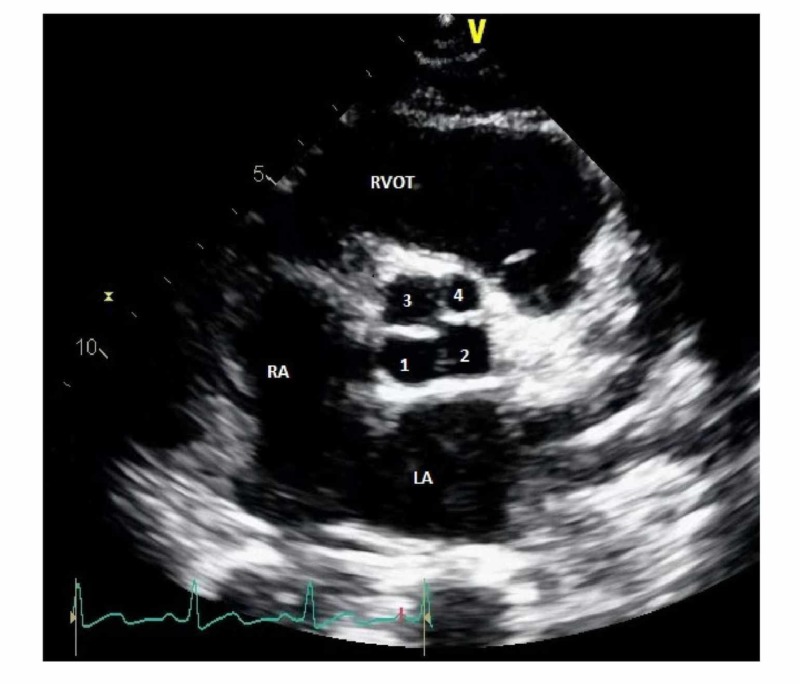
TTE showing the quadricuspid aortic valve Transthoracic echocardiogram (parasternal short axis) showing a quadricuspid aortic valve 1: noncoronary cusp; 2: left coronary cusp; 3: right coronary cusp; 4: accessory cusp; RA: right atrium; LA: left atrium; RVOT: right ventricular outflow tract
